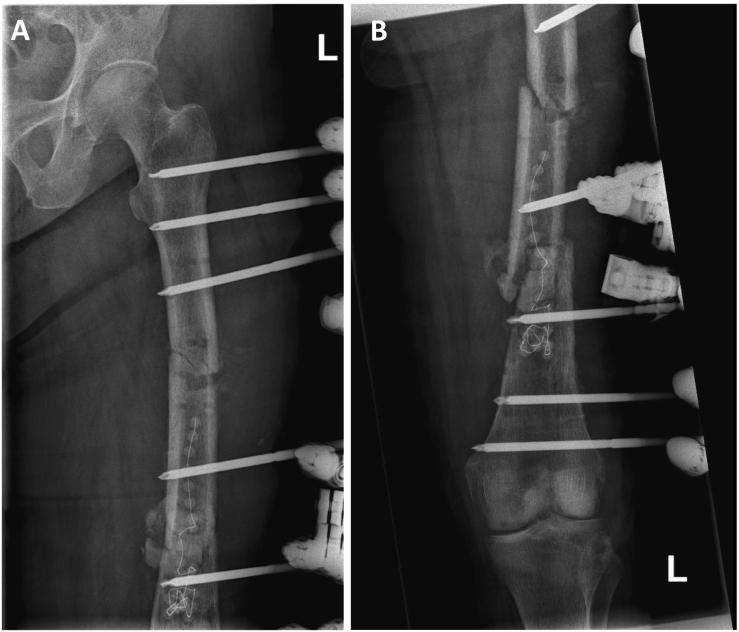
X-rays of the proximal (A) and distal femur (B) after fall with additional fresh fracture of the proximal femoral shaft and placement of additional pins into the proximal femur.
